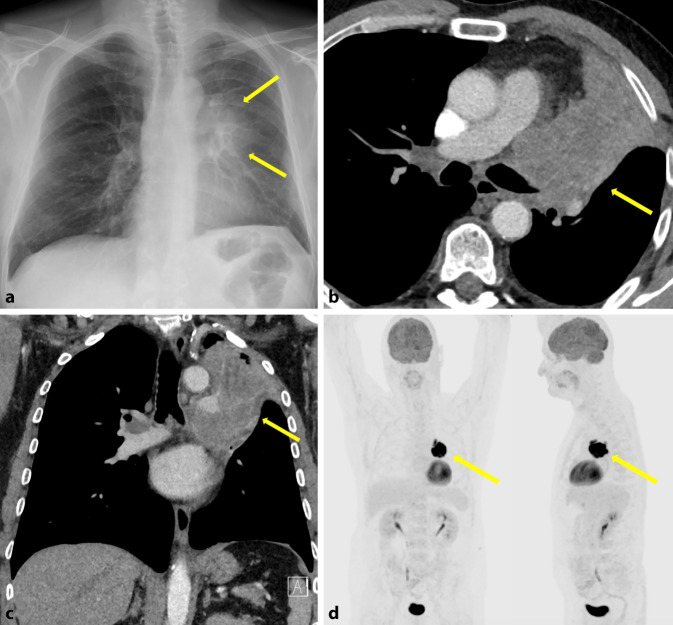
Die CT wird weithin als primäre Standard-Bildgebungsmethode zur Beurteilung des Nodalstatus eingesetzt. Sie ist jedoch unzuverlässig, wenn es darum geht, den Lymphknotenbefall allein anhand von Größenkriterien zu beurteilen. Die Sensitivität und Spezifität der CT für die Stadieneinteilung von Lungenkrebs sind relativ gering, nämlich nur 57 % bzw. 82 % [27]. Das Größenkriterium der morphologischen MRT ist ebenso genau wie die CT.
